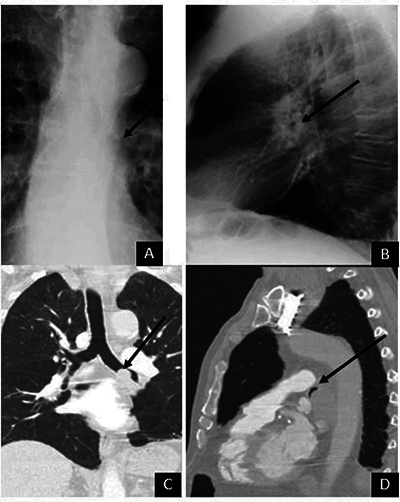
Chest radiography shows an AC (arrows) inside the lumen of the left mainstem bronchus on the lateral view (B) and an iceberg sign on the frontal view (A). Coronal (C) and sagittal (D) reformatted CT showing the bronchial tumour associated with an AC.
